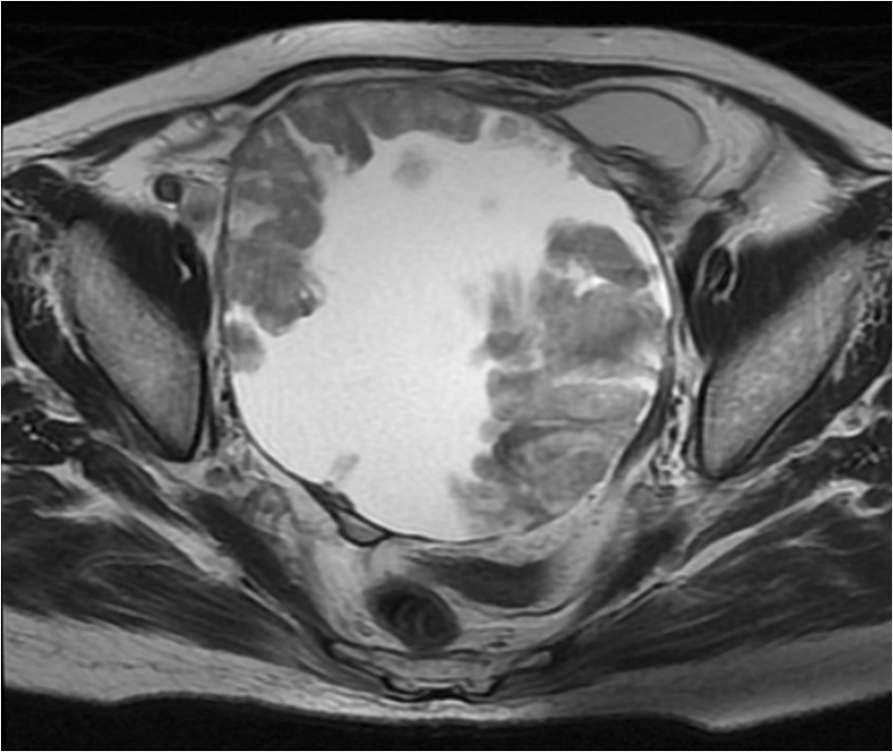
Pelvic magnetic resonance imaging. An axial T2-weighted image—of a 60-year-old woman presenting with an ovarian tumor—revealed a cystic mass with solid portions.
